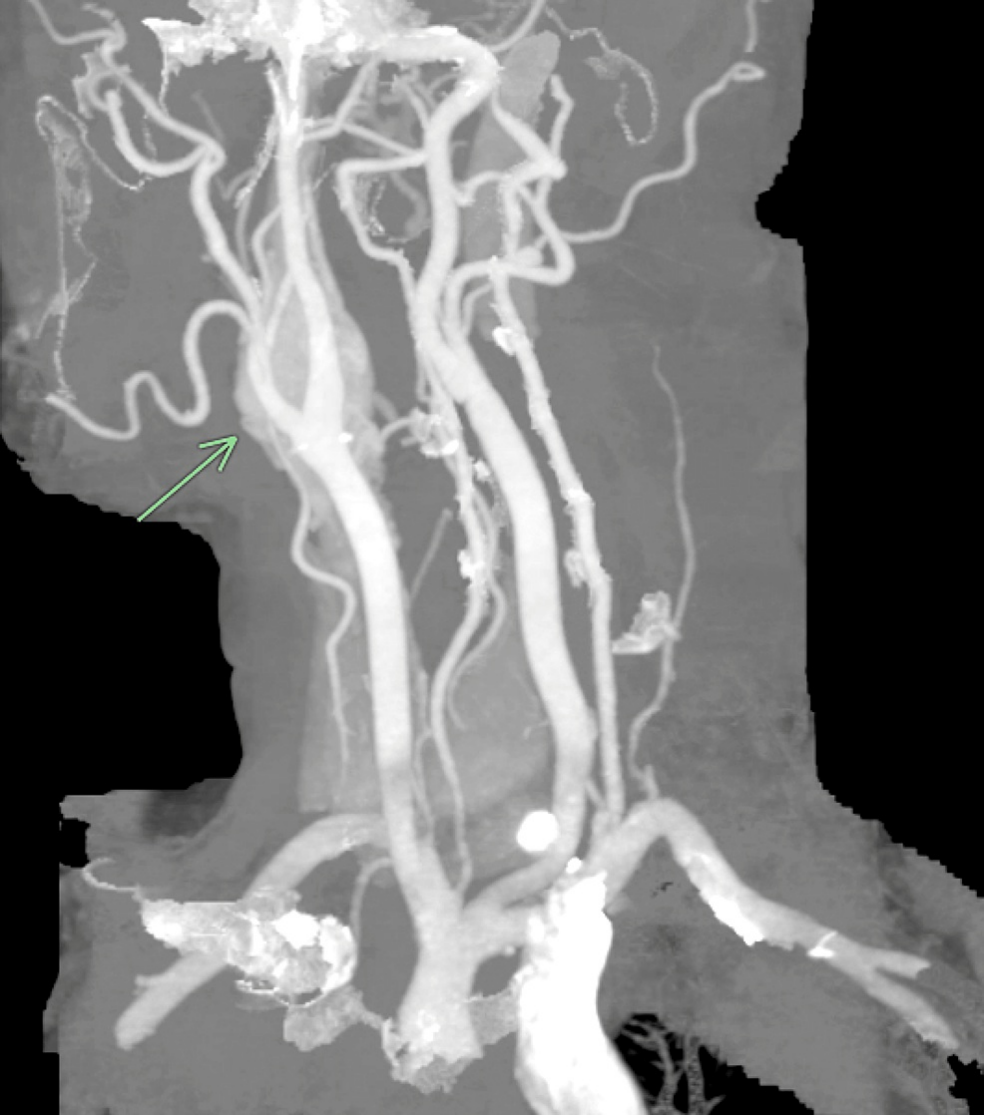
CTA of the neck demonstrating a left neck mass splaying the internal and external carotid arteries known as a Lyre sign
